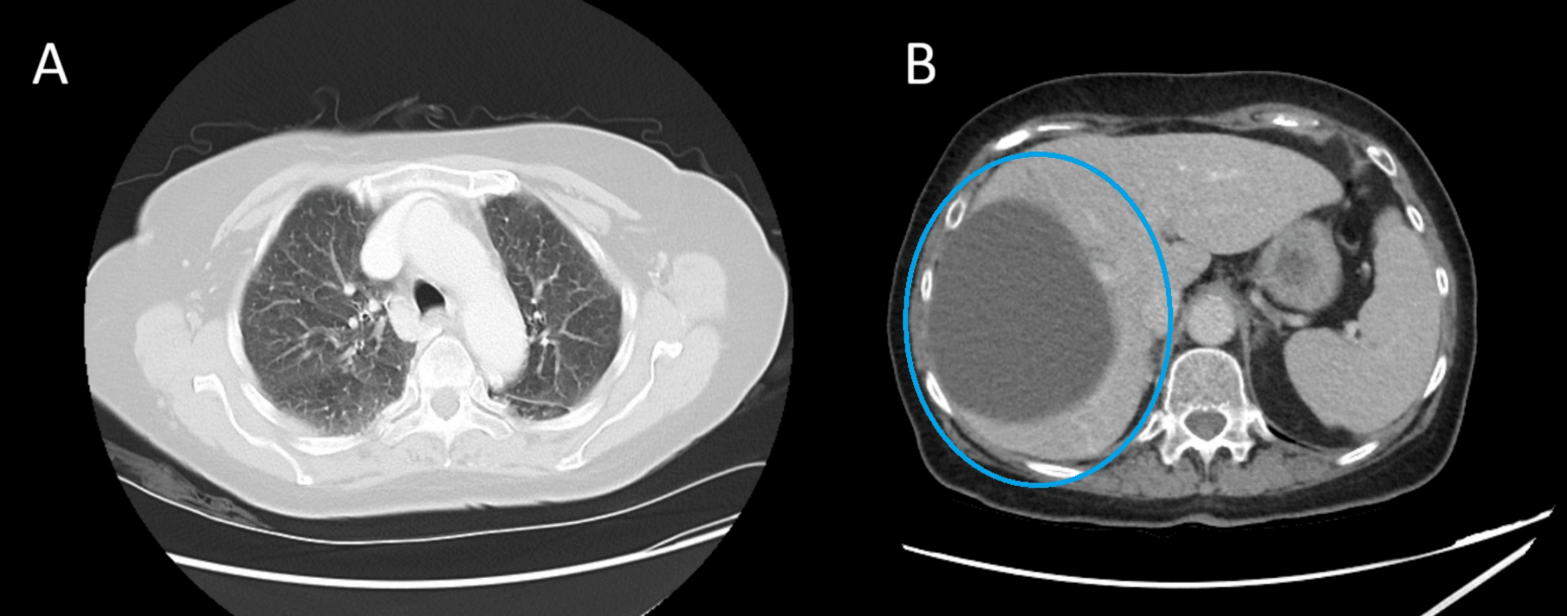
Representative contrast-enhanced chest and abdominal CT scan Contrast-enhanced axial CT images of the thorax and abdomen. (A) The thoracic section demonstrates normal lung fields with mild interstitial changes and no evidence of pulmonary embolism or focal consolidation. (B) The abdominal section reveals a large, well-defined, hypodense cystic lesion in the left hepatic lobe (circled in blue).
